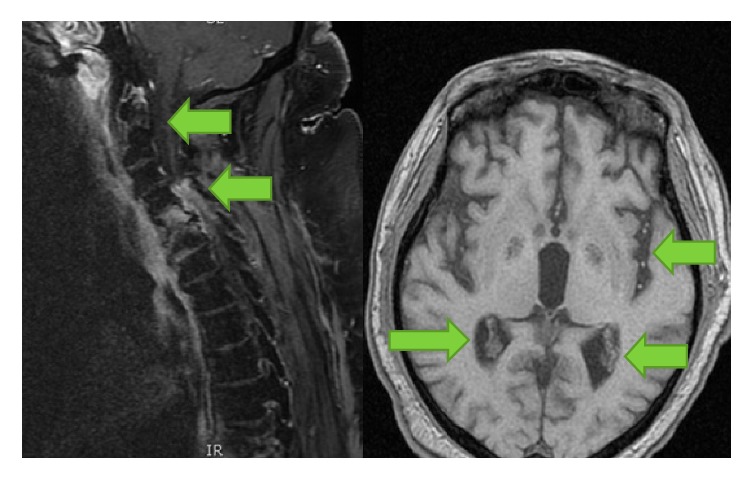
Cephalic and cervical MRI showing septic collections in cerebral ventricles and cervical spinal cord.
